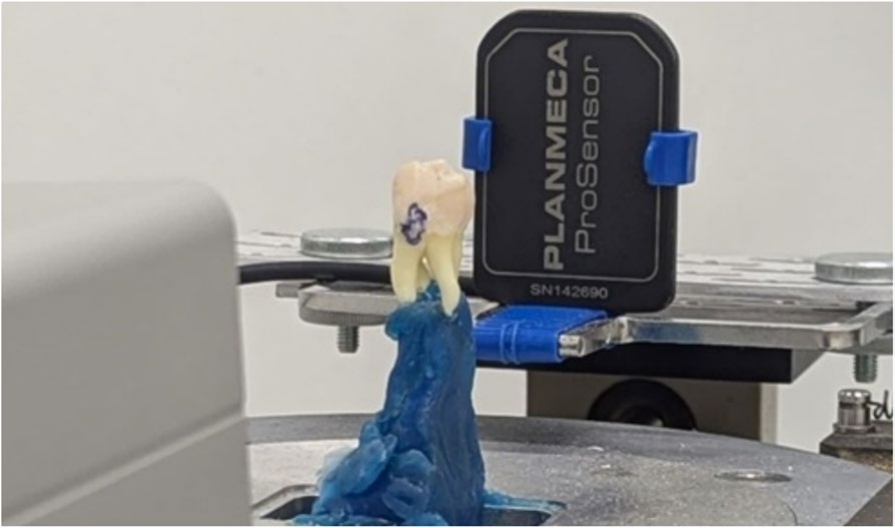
Setup for acquiring individual tooth radiographs
